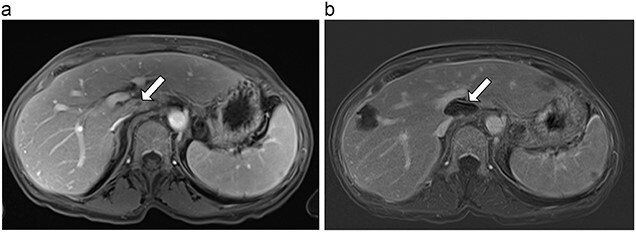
(a) Pre-ablation MRI demonstrating 5 mm lesion in caudate lobe of liver. (b) Post-ablation MRI (19 days postoperatively) demonstrating 36.2 mm complete ablation of caudate lesion.
